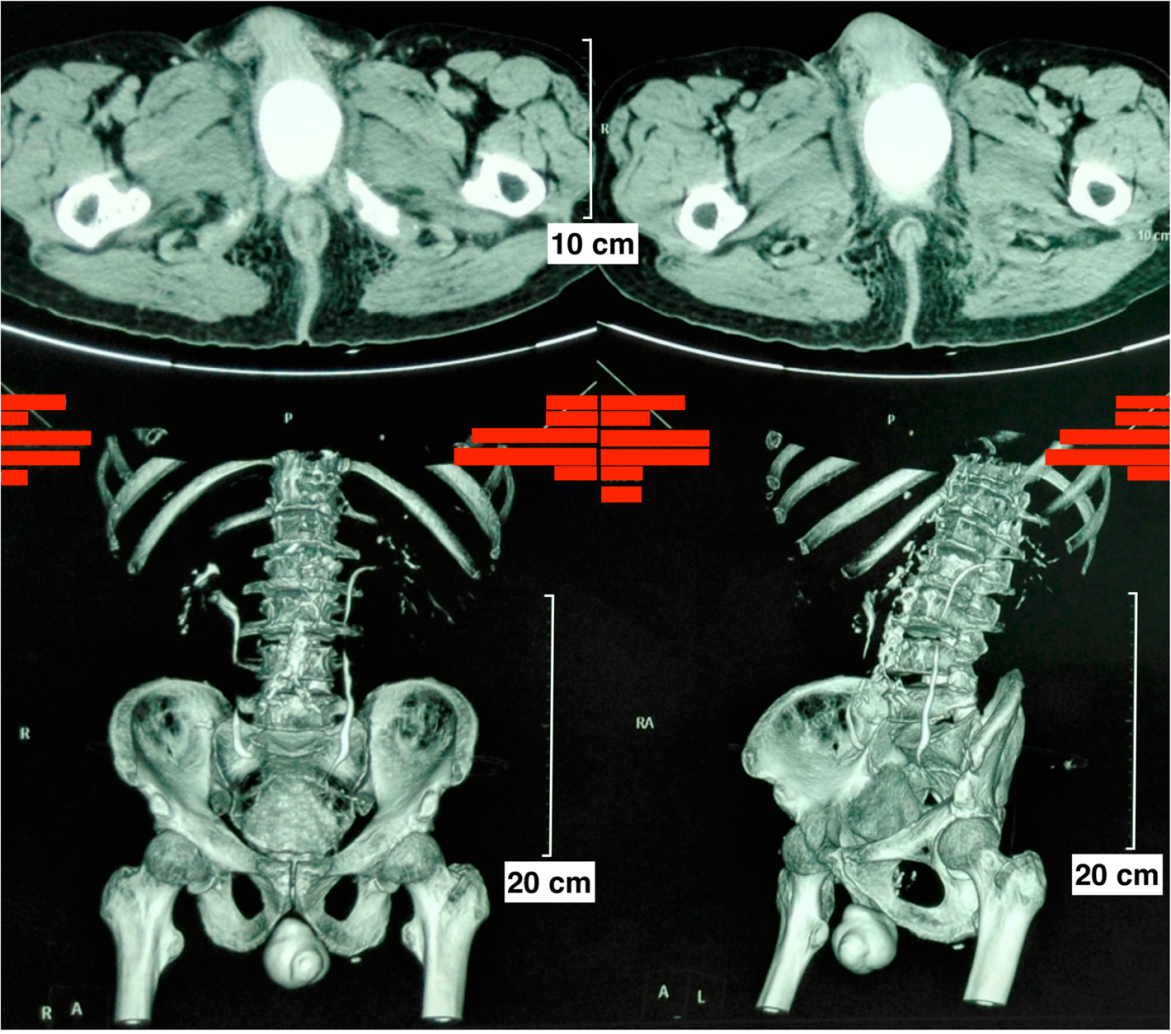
A giant urethral calculus with a size of 6.5 × 6 × 5.5 cm was observed below the pubic symphysis in the enhanced urinary CT
